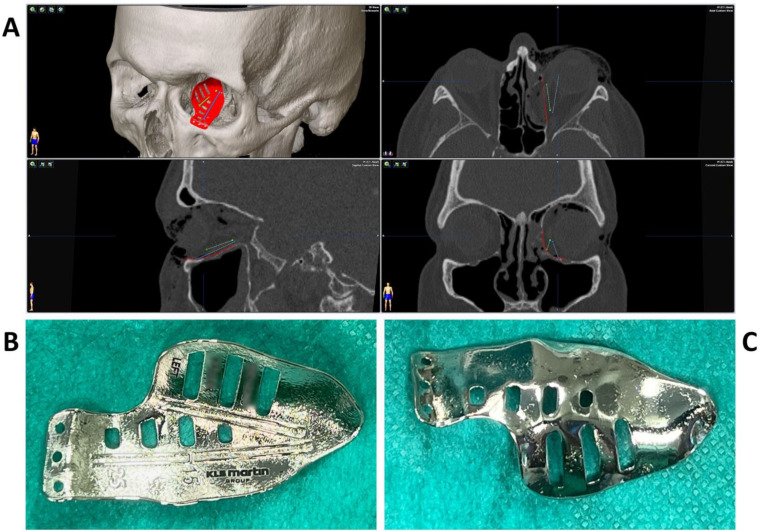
Multiplanar view of the pre-operative CT scan (A) with the finished computer-aided design of the patient-specific implant (red) for reconstruction of the damaged left orbit. Two trajectories (blue and green) have been defined which follow the grooves on the upper side of the implant (B) for position control via intraoperative navigation. The underside of the implant (C) shows a surface smoothened by plasma electrolytic polishing.
